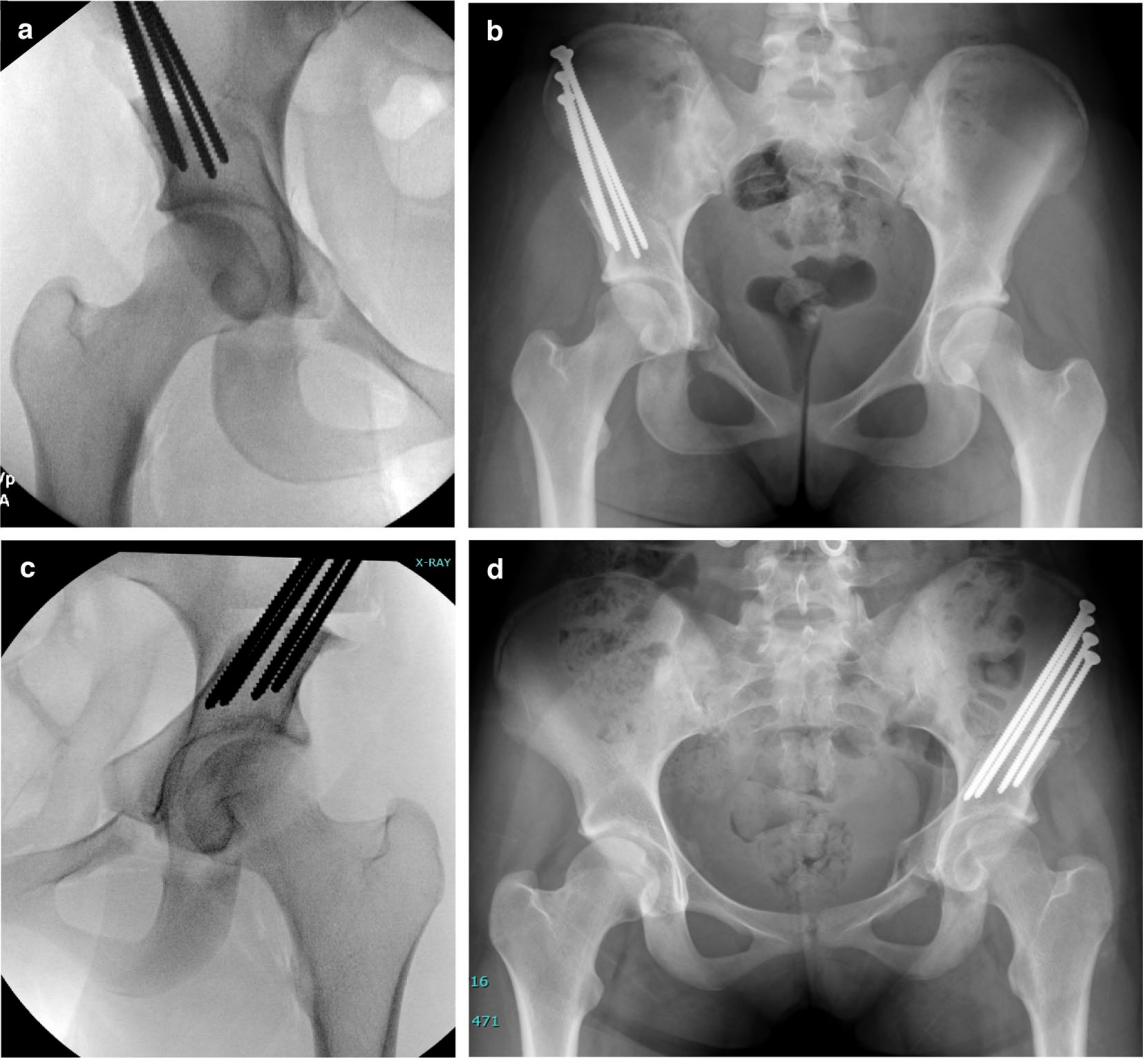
Intraoperative fluoroscopic images compared to their anteroposterior (AP) pelvis radiograph taken 6-weeks postoperatively. (a) Patient 1 image taken by the Standard Fluoroscope with an Image Intensifier intraoperatively. (b) Patient 1 AP pelvis taken six-weeks postoperatively. (c) Patient 2 image taken intraoperatively by the novel Fluoroscope with Flat Panel Detector Fluoroscope. (d) Patient 2 AP pelvis taken six-weeks postoperatively.
